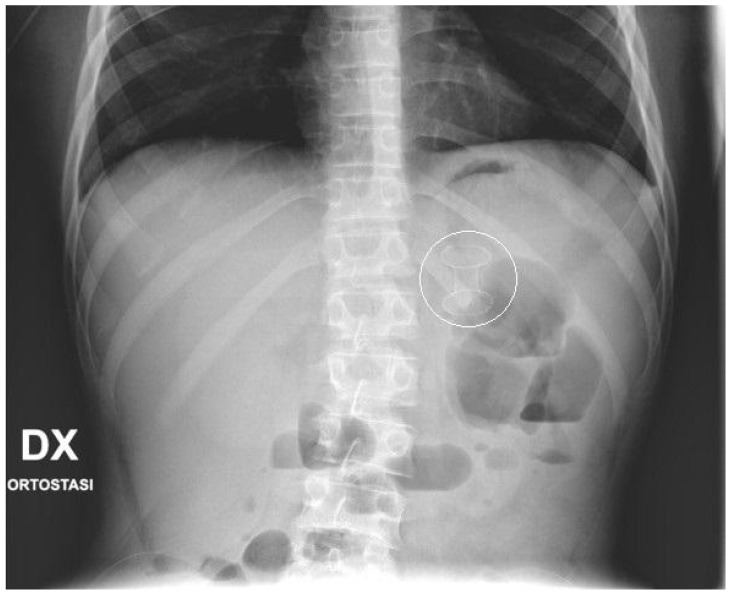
Final X-ray. X-ray performed at the end of the procedure that shows the EC-LAMS as a radiopaque dumbbell-shape object projected in the epigastrium.
